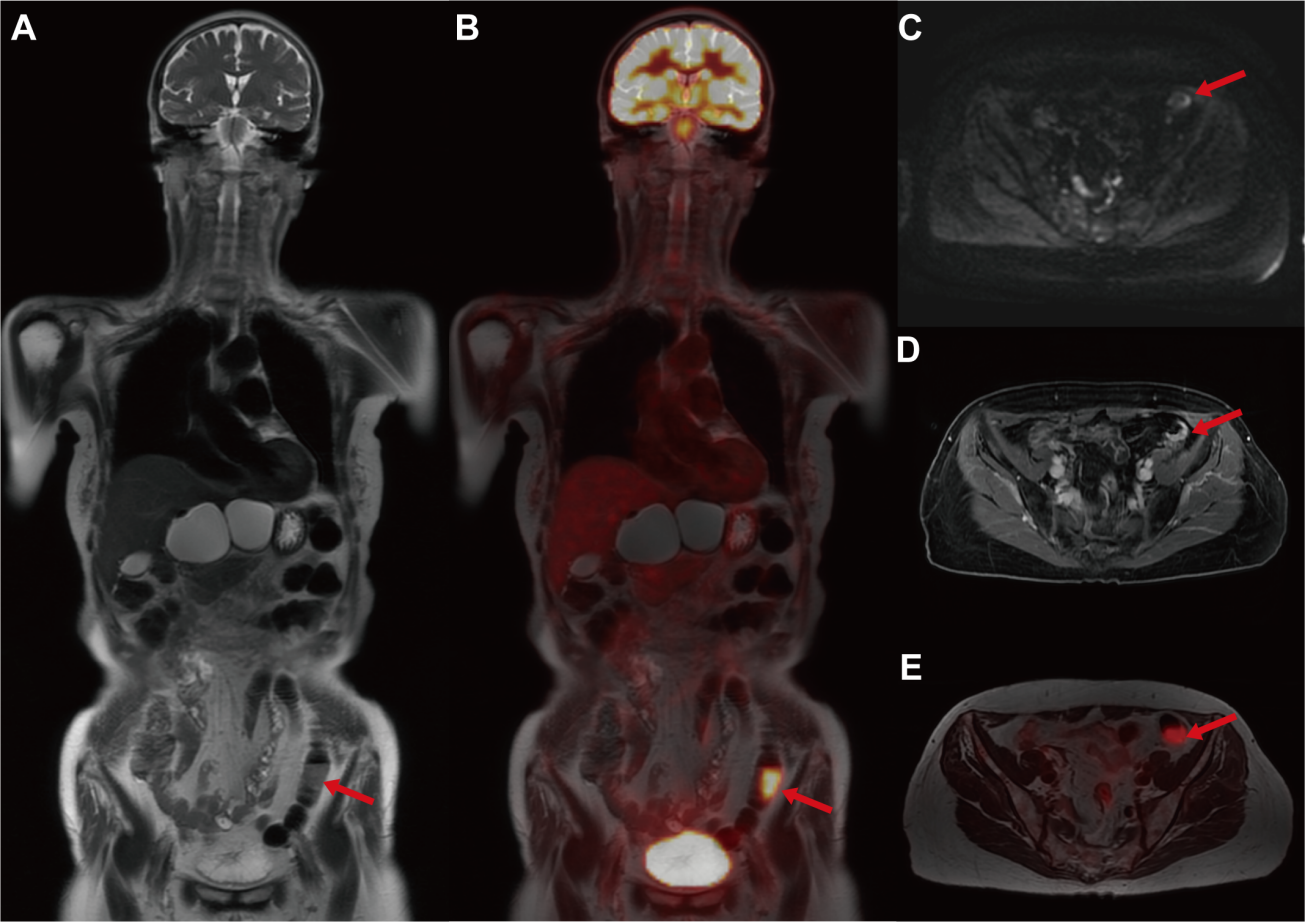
The multimodal 18F-FDG PET/MR images for evaluating the descending colon mass. (A) Coronal whole-body T2WI. (B) Coronal PET/T2WI fusion image. (C) Axial DWI. (D) Axial T1WI+C. (E) Axial PET PET/T2WI fusion image. The coronal whole-body T2WI and PET/MR fusion images showed that the abnormal hypermetabolism on PET images was a mass in the intestinal wall of the descending colon (red arrow). The thickened descending colon wall that showed markedly high signal intensity on either DWI (ADC_mean_ = 0.75*10^-3 mm2/s) or T1WI+C and hypermetabolism on axial PET/MR fusion images was observed (red arrow).
